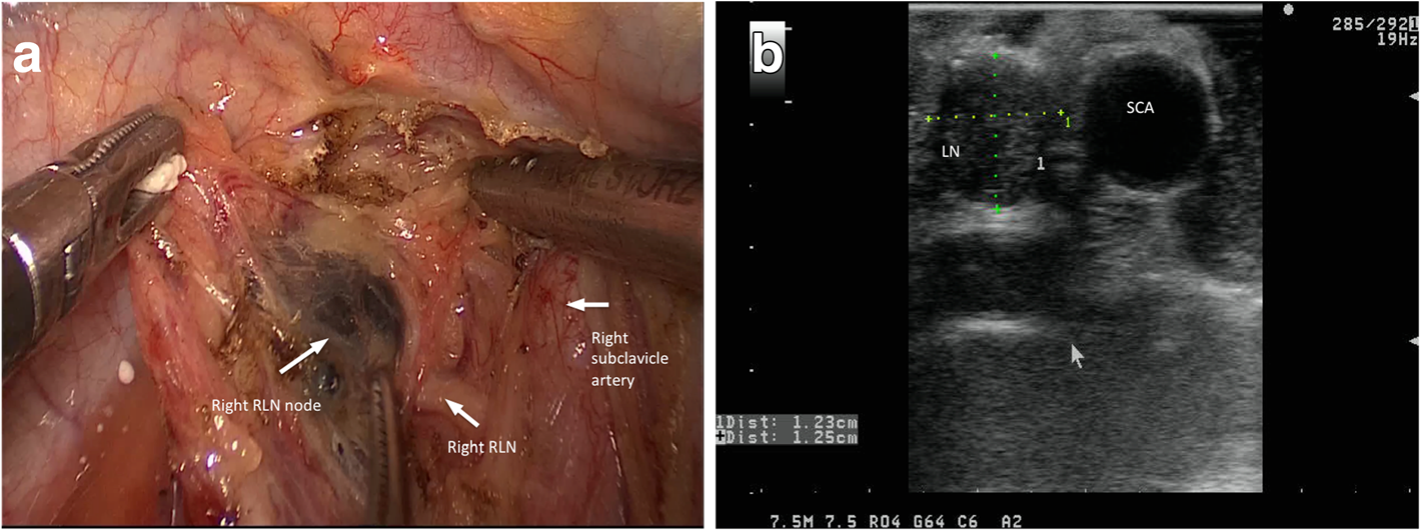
a Right thoracic recurrent laryngeal nerve (RLN) node dissection. b Intraoperative ultrasound image showed the metastatic lymph node 1.25 cm in diameter located in the right RLN region. SCA, subclavicular artery
